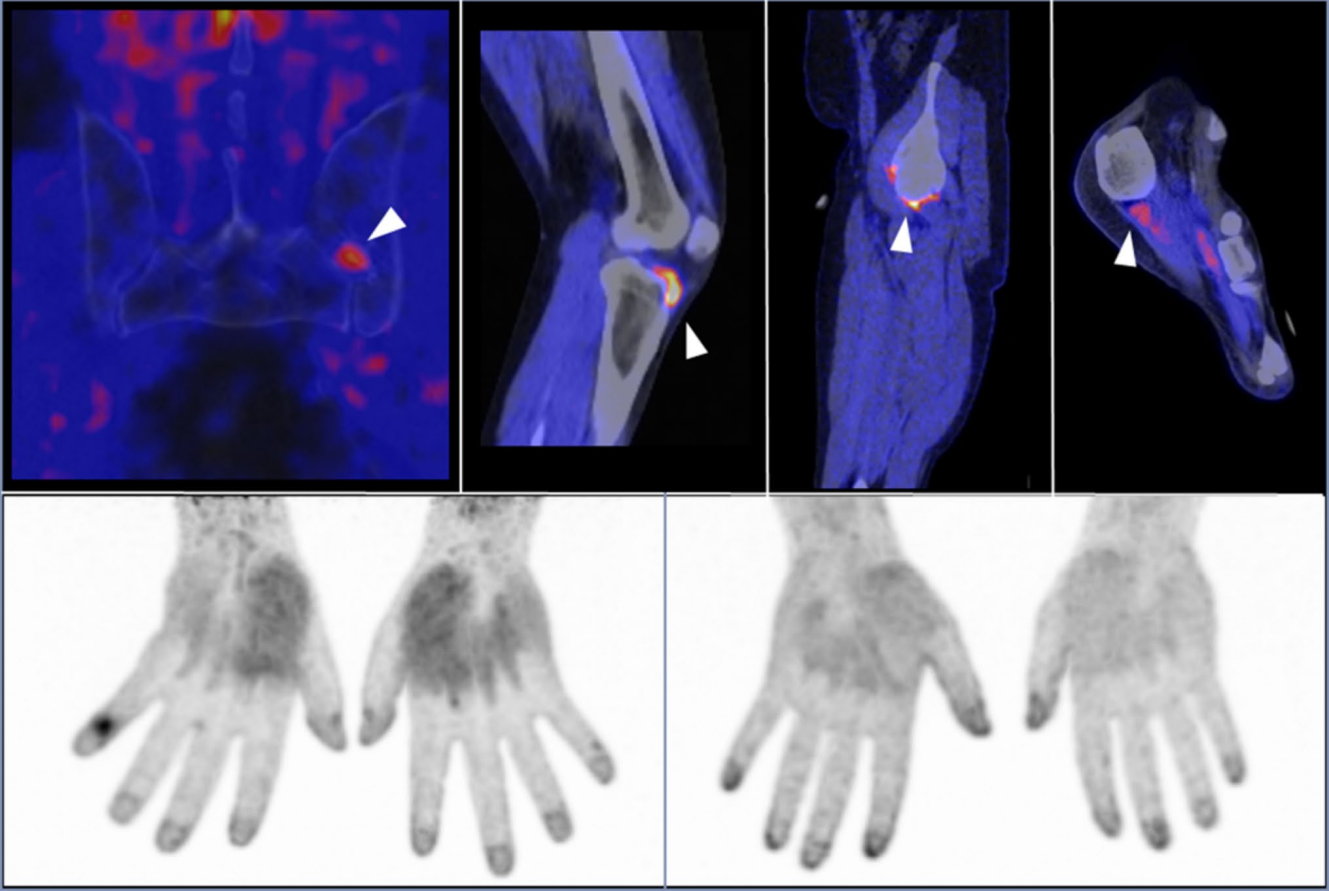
^68^ Ga-FAPI-PET/CT imaging of patients with active Psoriatic Arthritis. Upper panel (left to right): sacroiliac joint FAPI uptake in a PsA patient with sacroiliitis, FAPI-positive synovitis with effusion in the knee joint, synovial FAPI uptake in the hip joint during monolateral coxitis, enthesitis of the plantar fascia with perientheseal FAPI uptake. Lower panel (left to right): DIP joint arthritis of the 5th right finger with correspondent FAPI signal increase, FAPI tracer accumulation at the periungual region of all fingers in the context of active nail psoriasis
